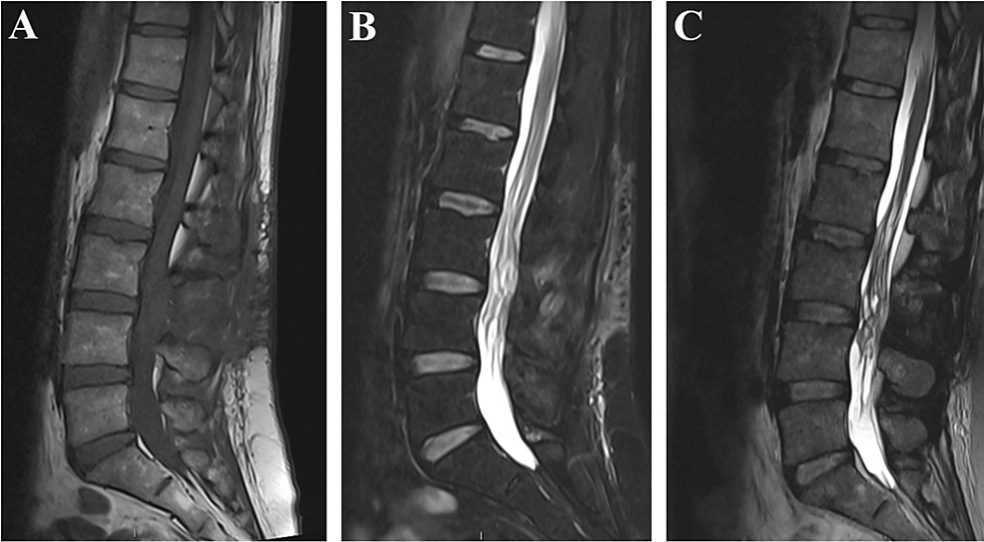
Three-month postoperative MRI A: Sagittal T1-weighted MRI of the spine. B: Sagittal T2-weighted Dixon water MRI of the spine. C: Sagittal three-dimensional SPACE T2 STIR MRI. MRI: magnetic resonance imaging, STIR: short-TI inversion recovery, SPACE: sampling perfection with application-optimized contrasts using different flip angle evolution
